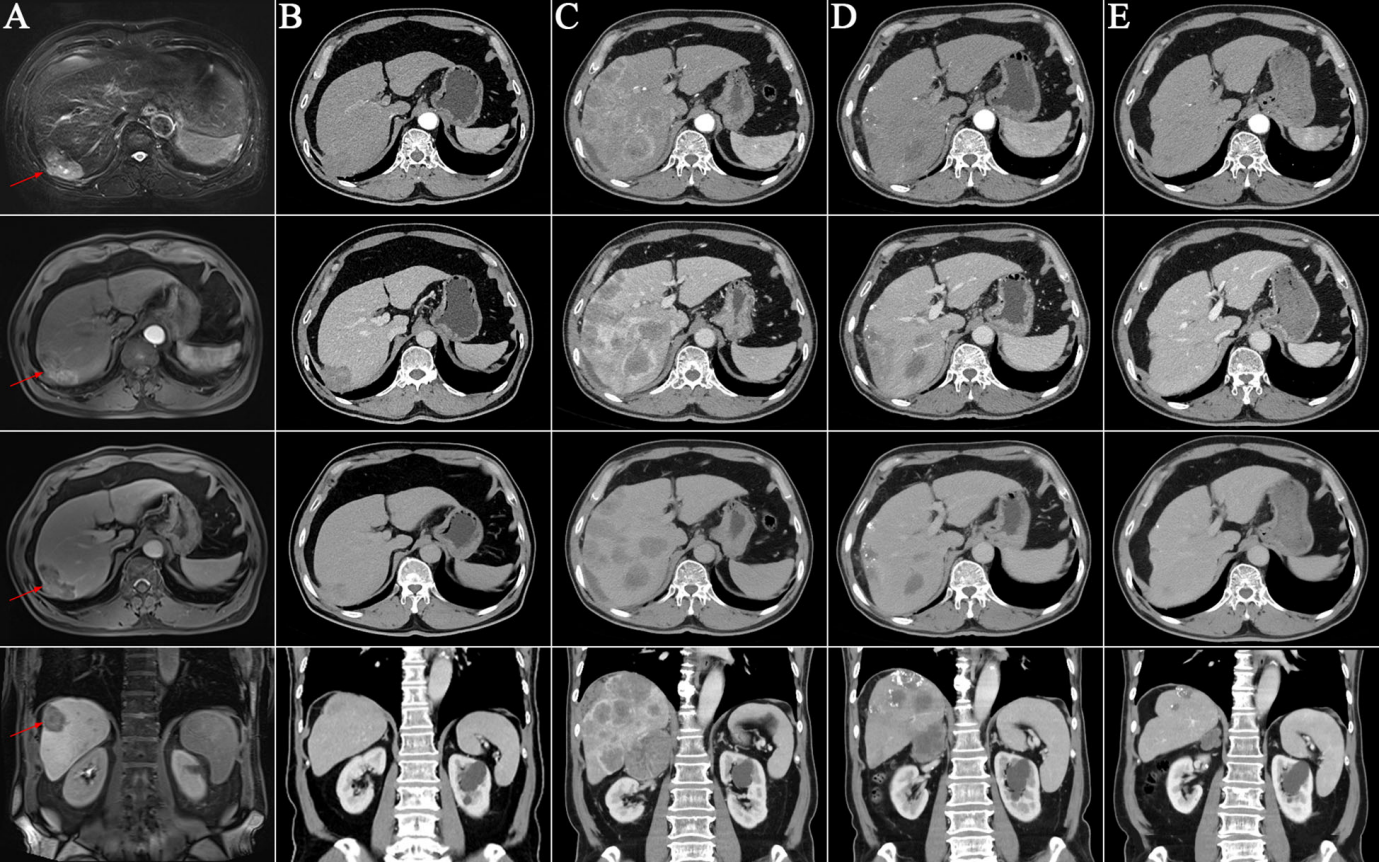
Enhanced liver imagings at different time points. (A) The T2-phase, arterial phase, portal vein phase, and coronal image of the enhanced liver magnetic resonance imaging on May 26, 2020. It revealed a mass in the right hepatic lobe (red arrows); (B-E) The arterial phase, portal vein phase, equilibrium phase and coronal image of the enhanced liver computed tomography images; (B) No signs of tumor on October 21, 2021; (C) Diffuse recurrence in the liver on May 7, 2022; (D) After two cycles of retreatment of immunotherapy on July 21, 2022. Significant reduction of liver lesions; (E) CT indicated no enhancing tumor lesions in the liver on September 15, 2023.
